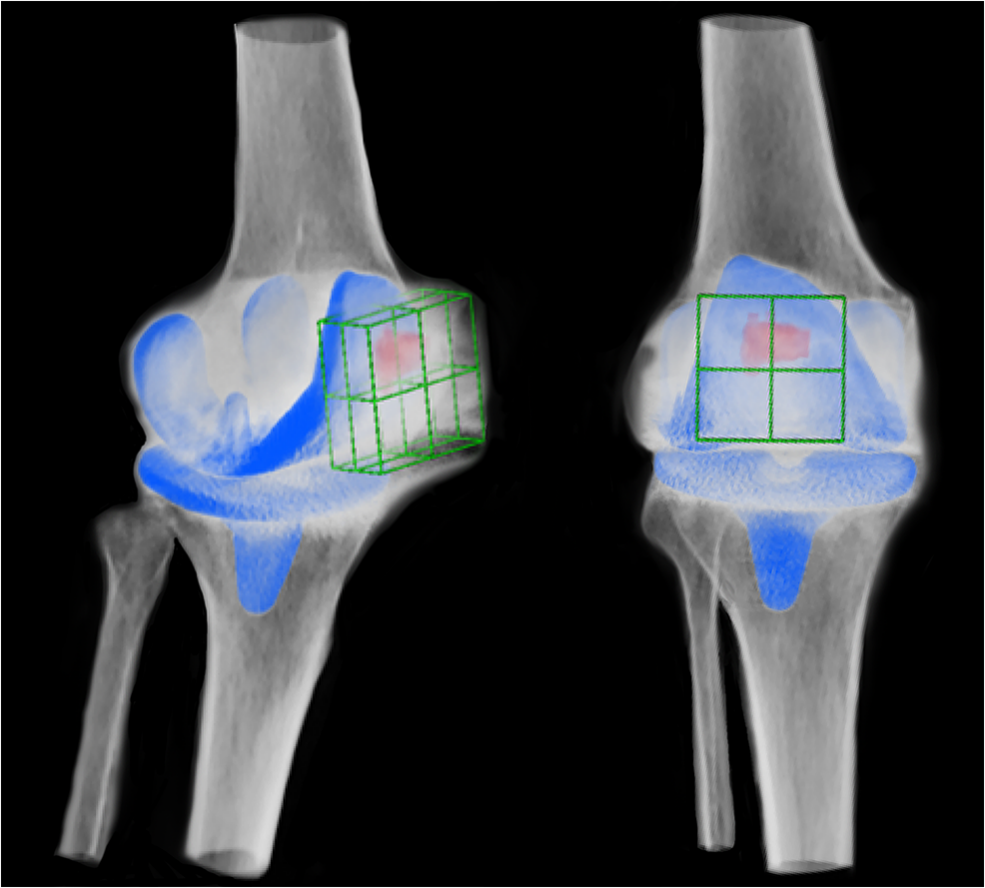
Three-dimensional reconstructed SPECT/CT images. An 8-quadrant patellar grid was used to measure and localise the in vivo bone stress using a customised software (OrthoExpert©, London, UK)
